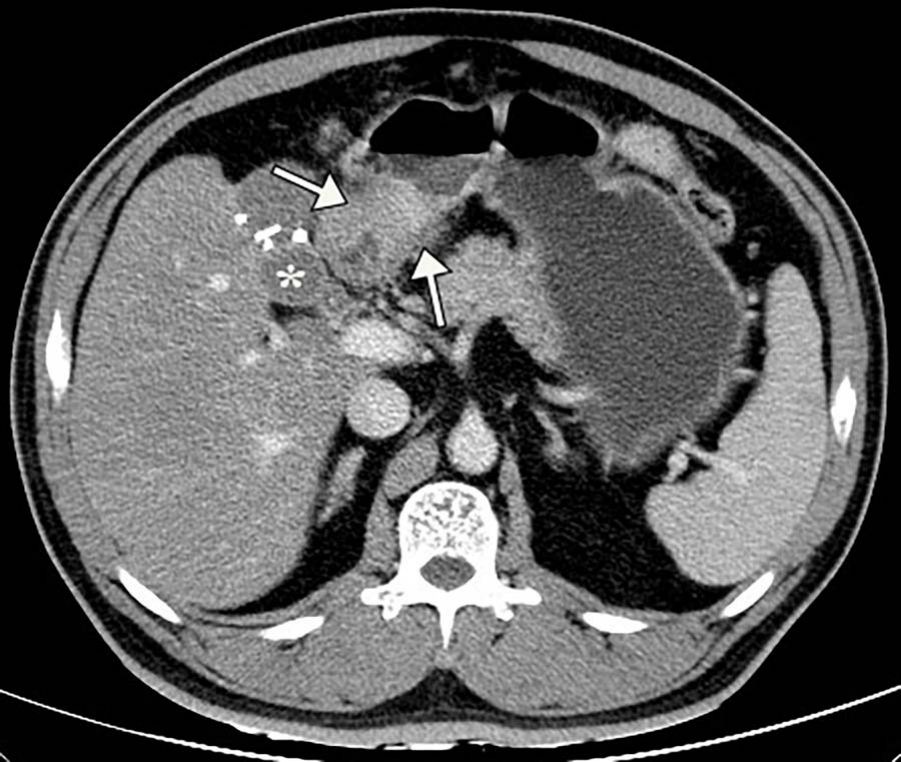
Preoperative contrast abdominal CT scan showed the thickening antral wall with uneven enhancement (white arrows) and a cystic foci (white asterisk) in the gallbladder bed with hyperdense clips from previous cholecystectomy.
